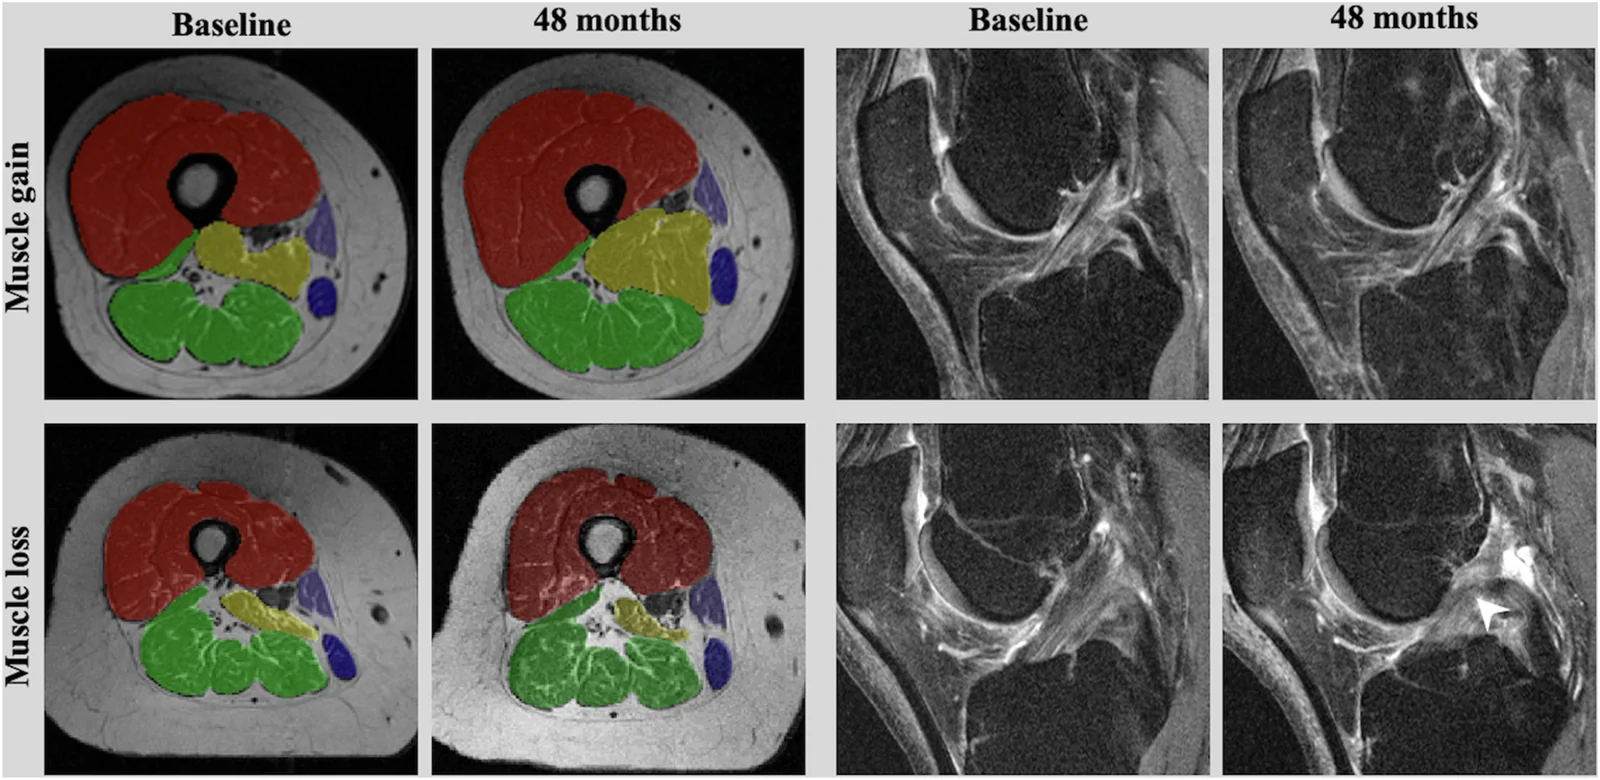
Imaging examples of changes in anterior cruciate ligament (ACL). Left: Axial T1-weighted MR images of the right thigh with artificial intelligence-enabled muscle segmentation at baseline and after 48 months. Right: Sagittal intermediate-weighted fast spin-echo fat-suppression sequence of the right knee at baseline and after 48 months. The patient with muscle gain (predominantly of the adductors (yellow) and quadriceps (red), with an approximately 6% increase in total muscle volume over 48 months) was a 53-year-old woman without knee pain at baseline and at 48 months (WOMAC pain subscores of 0) showed no change of the ACL (WORMS ACL subscore of 1 for both at baseline and at 48 months). The patient with muscle loss (predominantly of the quadriceps (red), with an approximately 9% decrease in total muscle volume) was a 62-year-old man with mild knee stiffness at baseline and at 48 months (both with a WOMAC stiffness subscore of 2) showed a new partial tear of the ACL after 48 months (white arrowhead), with baseline WORMS ACL subscore of 0 and 3 at 48 months.
